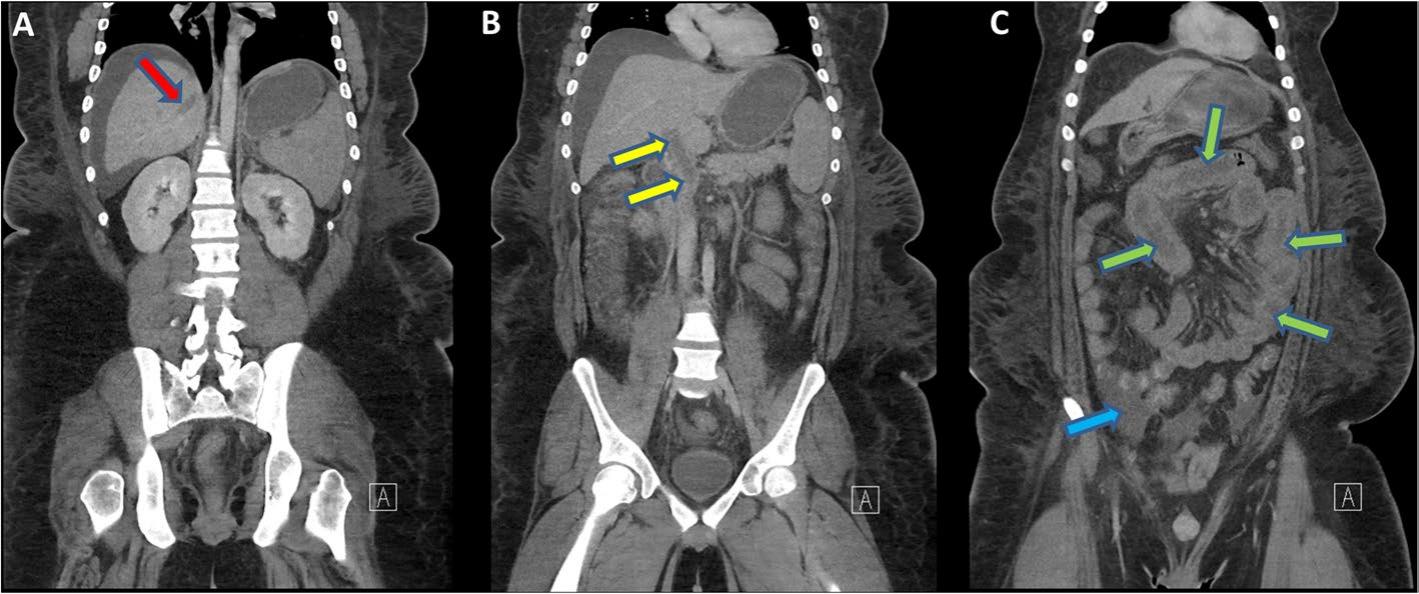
Coronal CT reconstruction showing thrombosed right hepatic (red arrow) and portal veins (yellow arrows), congested small bowel (green arrows) and small ascites (blue arrow)
